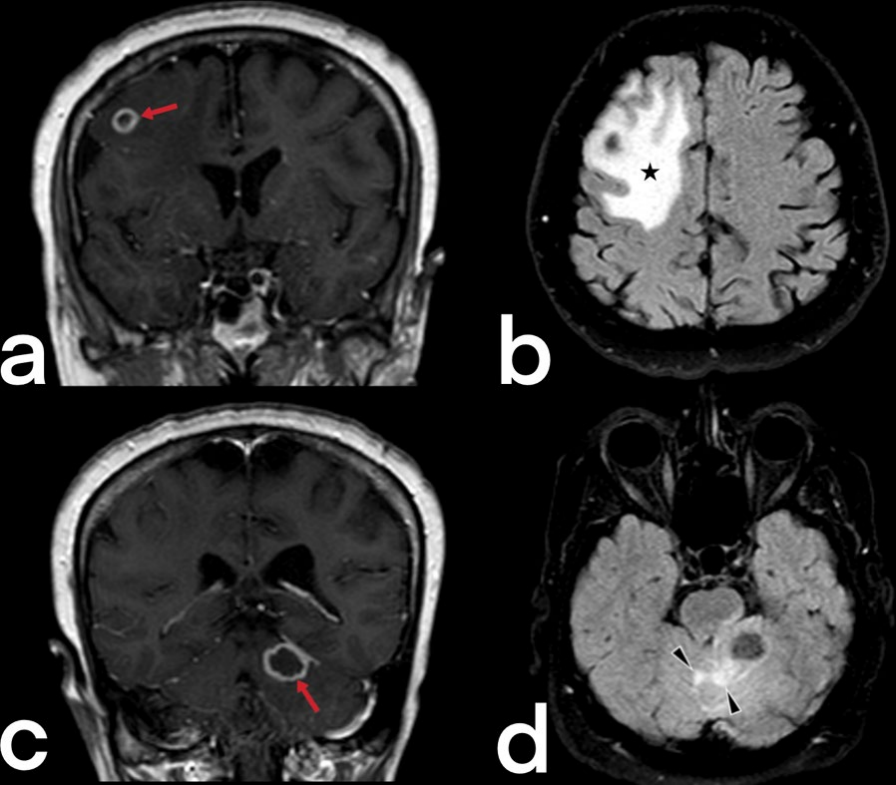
MR images of a 63-year-old female with central nervous system TB. Focal lesions (arrows) with rim-like enhancement are seen (tuberculoma) on contrast-enhanced coronal T1 weighted images (a, c). Perilesional increased signals (asterisk and arrowheads) due to vasogenic edema are noted on axial FLAIR images (b, d)
